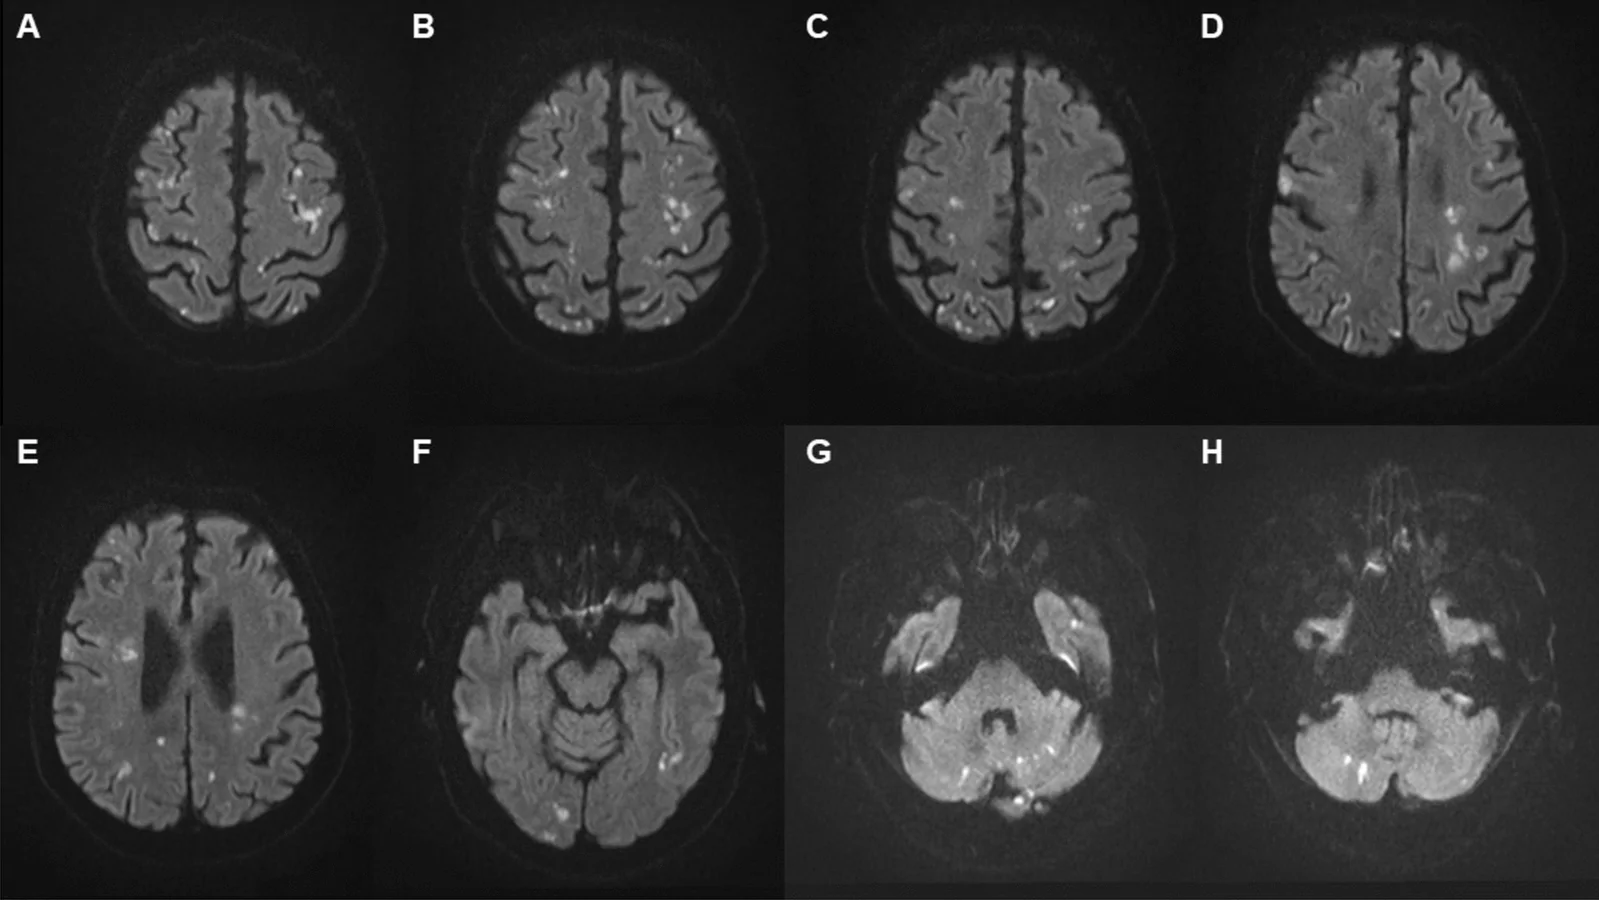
A-E. Diffusion-weighted axial cranial magnetic resonance images, A-E multiple bihemispheric cortical lesions affecting the anterior circulation; F lesions in the posterior circulation; G, H cerebellar involvement
